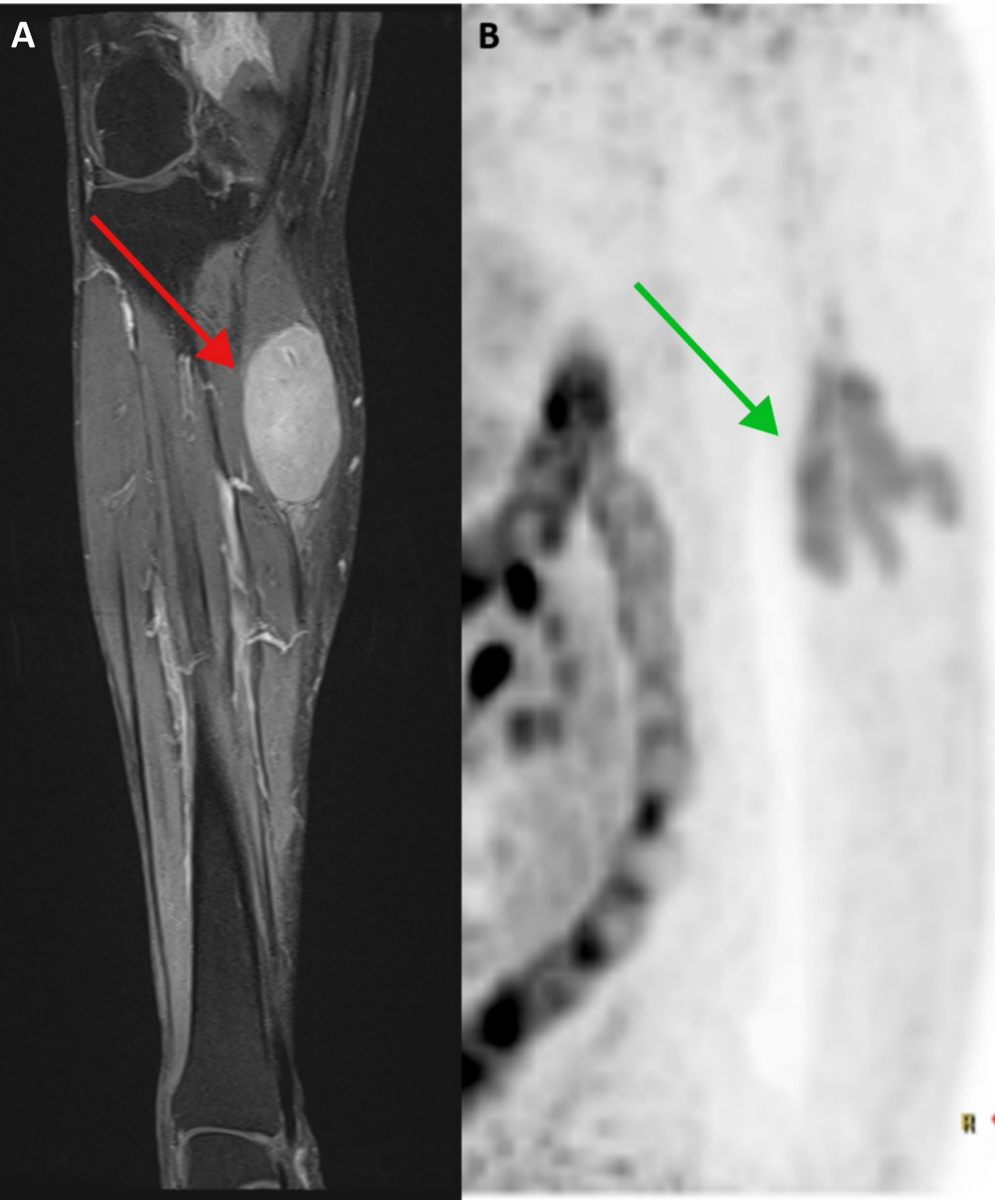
Soft tissue mantle cell lymphoma tumors. (A) Case 1: MRI with contrast of right tibia and fibula demonstrates oval well-demarcated T1 isointense, homogeneously enhancing mass within the substance of the proximal medial gastrocnemius muscle (red arrow); (B) Case 3: PET-CT demonstrating an FDG-avid soft tissue mass in the medial left elbow (green arrow)
